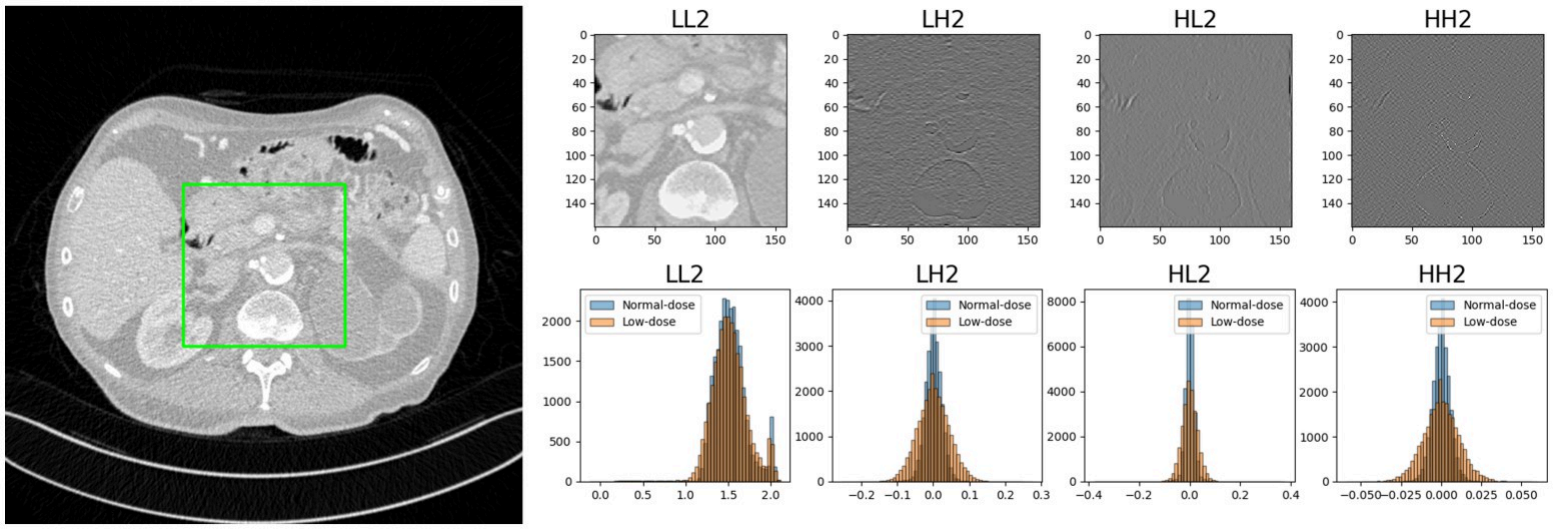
Example of a stationary wavelet transform (above) and comparison of the histogram distribution (below) of low-dose and normal-dose (reference) CT images in stationary wavelet transform domain. For better visuality, we included only *LL*_2_, *LH*_2_, *HL*_2_, and *HH*_2_ subbands. Four different images correspond to histograms for the low-frequency (*LL*_2_) and high-frequency subbands (*LH*_2_, *HL*_2_, and *HH*_2_).
